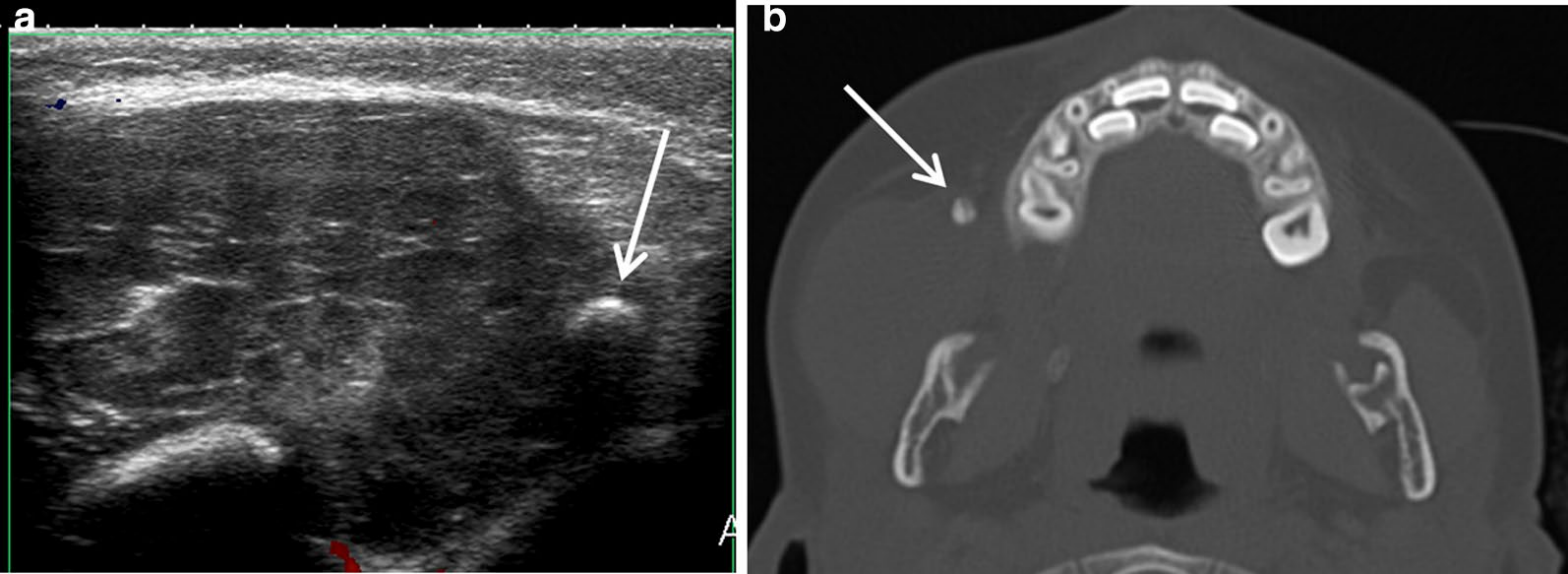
Venous malformation in the right masseter, with a typical phlebolith, on ultrasonography (a) and axial CT image (b)
